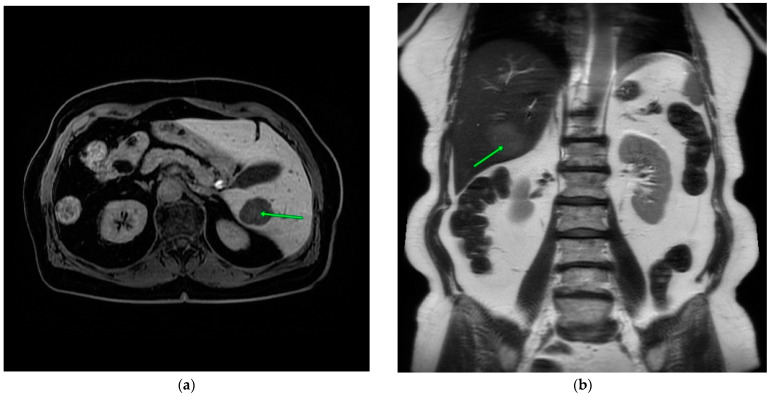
Abdominal MRI. Green arrows point the lesion (a,b).
